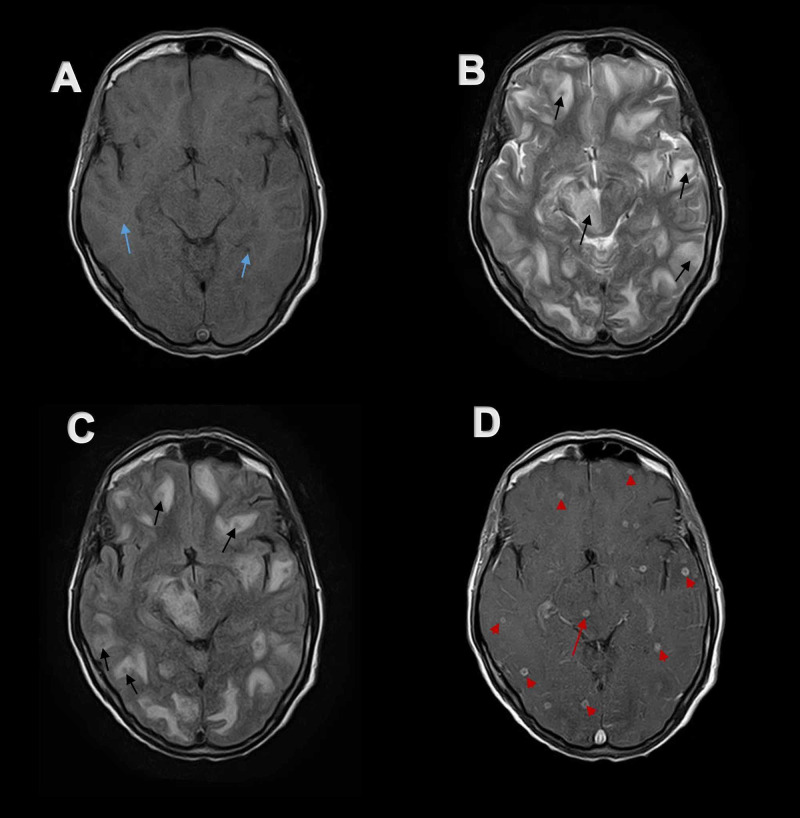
Axial MR imaging of the brain at the level of midbrain Multiple small focal lesions are dispersed throughout the brain. (A) Lesions appear isointense to hypo-intense (blue arrows) on axial T1-weighted MR image. (B and C) These lesions demonstrate hypo-intense signal (black arrows) on axial T-2 weighted MR image and axial FLAIR MR image. (D) Same lesions show peripheral contrast uptake on post-contrast axial T1-weighted MR image with central hypo-intense signal (red arrow heads) demonstrating ring enhancement. A single lesion in the right midbrain (red arrow) is also visible. MR, magnetic resonance. FLAIR, fluid-attenuated inversion recovery
